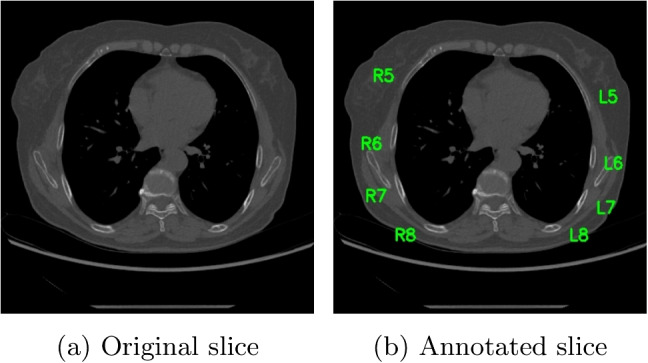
An axial chest CT slice and slice with rib annotations. The original slice requires repetitive investigation for identifying rib numbers among hundreds of CT slices to designate abnormal region, whereas annotated slice enables radiologists to skip superfluous procedure
